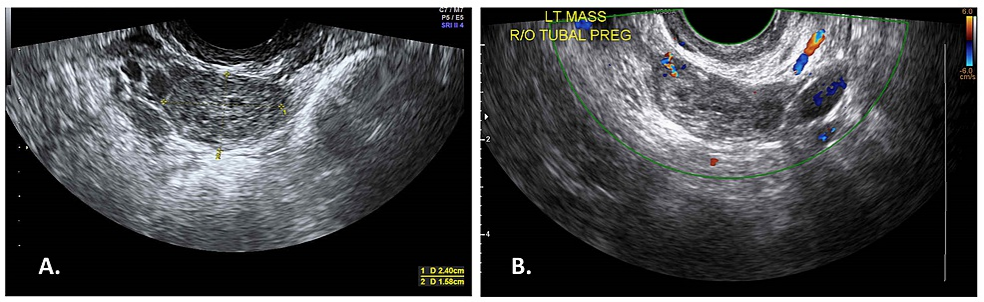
TVUS on the first OPD day and initial MTX day. A: TVUS on the first OPD day. A left tubal ectopic mass measuring 2.4 x 1.58 cm was observed. There was no yolk sac or fetal pole formation. B: TVUS on the day of initial MTX treatment (nine days after first OPD). The left tubal ectopic mass measured 2.3 x 1.3 cm. TVUS: transvaginal ultrasonography; OPD: outpatient department; LT: left. R/O: rule out. PREG: pregnancy.
